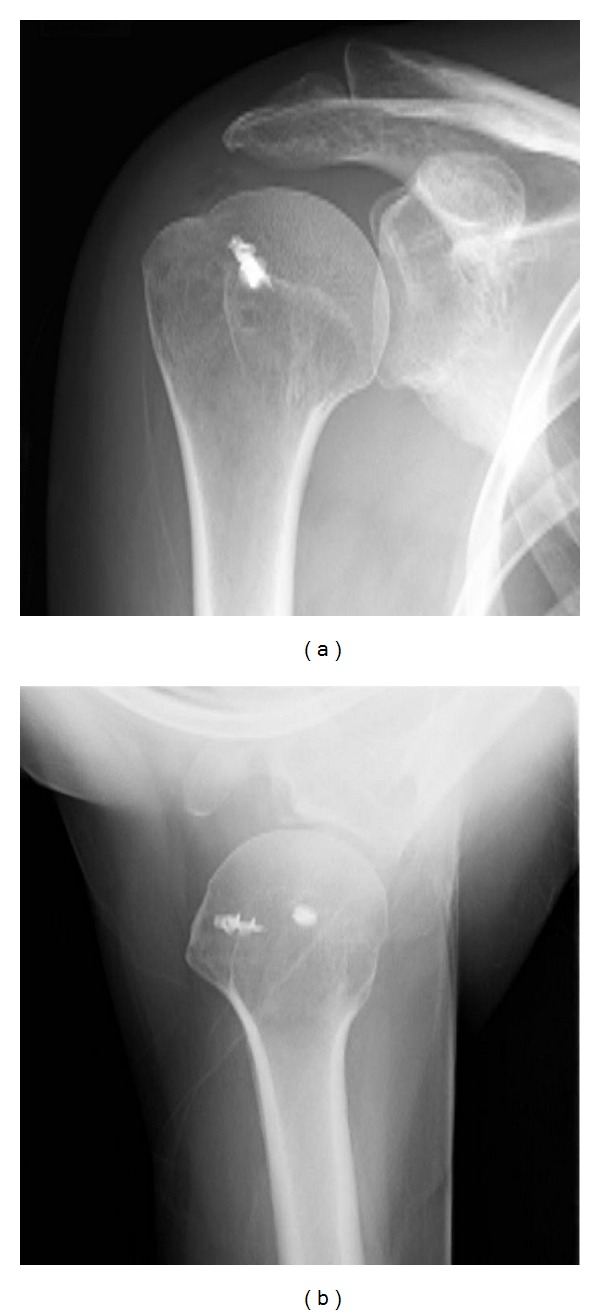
(a) and (b) Postoperative radiographs (anteroposterior view, axillary view) showing the subsidence of the calcific deposits.
